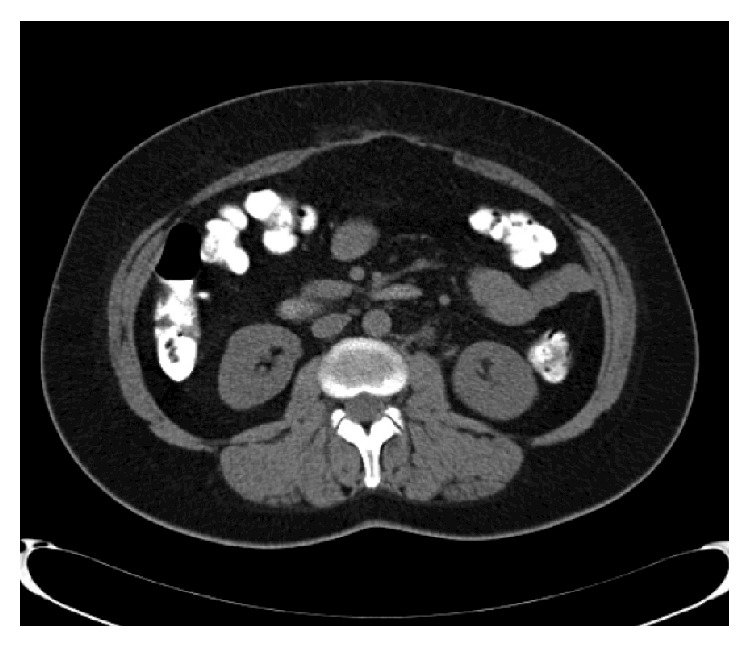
Abdominal computed tomography (CT) scan one year after the operation; the postoperative site is free of recurrent disease.
